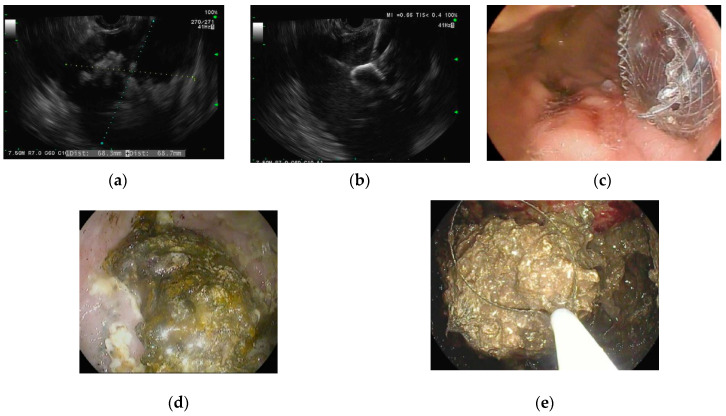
Endoscopic ultrasound-guided drainage using a LAMS with subsequent direct endoscopic necrosectomy for walled-off pancreatic necrosis (WOPN) (a) Endoscopic ultrasound imaging of cyst cavity with necrotic debris; (b) endoscopic ultrasound-guided placement of LAMS into WOPN; (c) endoscopic imaging post LAMS deployment in the stomach; (d) endoscopic imaging inside cyst cavity with necrotic material; and (e) direct endoscopic necrosectomy performed using a snare.
